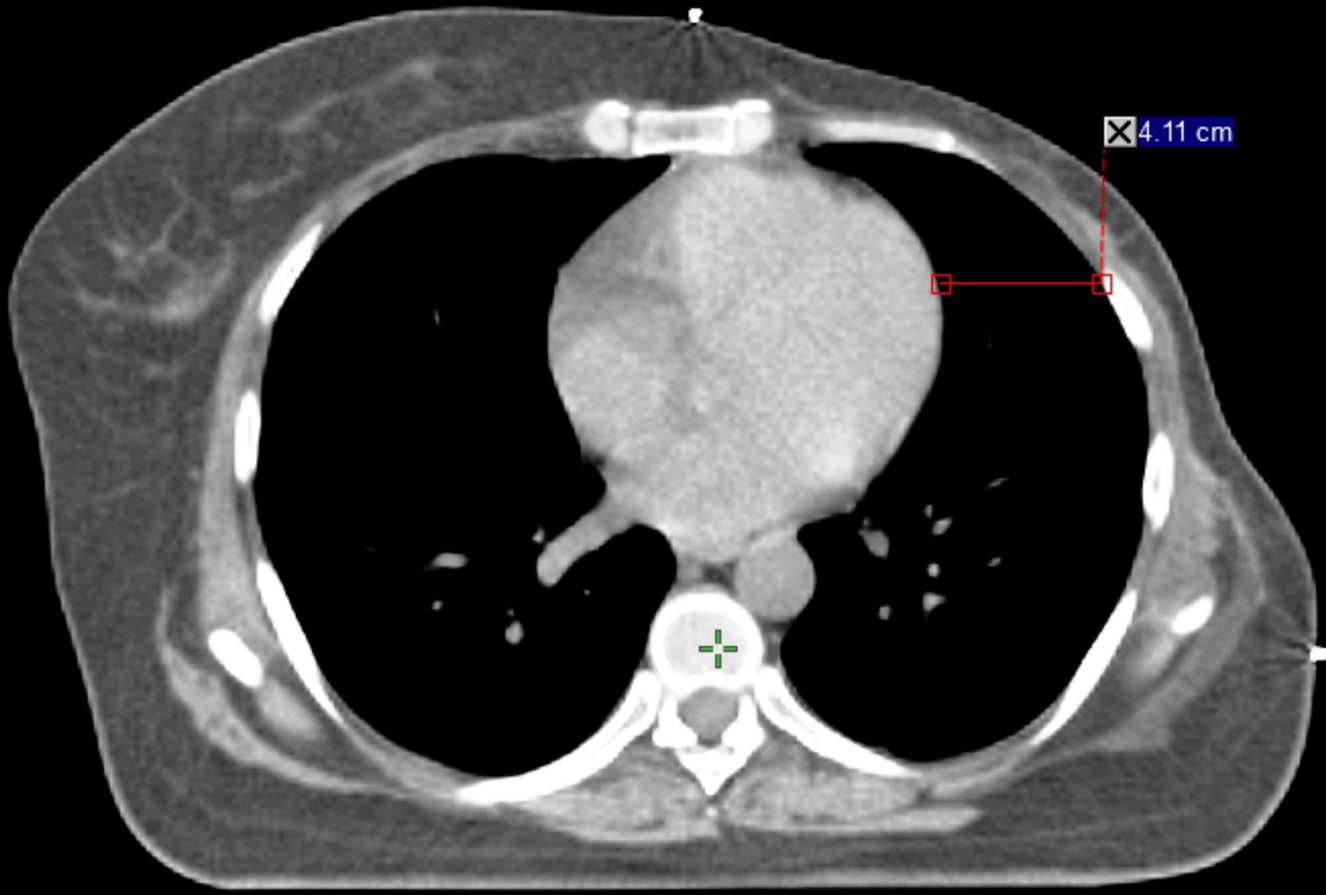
CT image acquired during vDIBH. This axial planning CT shows an increased separation of 4.11 cm between the anterior surface of the heart and the chest wall, demonstrating the effectiveness of vDIBH in displacing the heart posteriorly and inferiorly, away from the tangential radiation fields. vDIBH: voluntary deep inspiration breath hold
